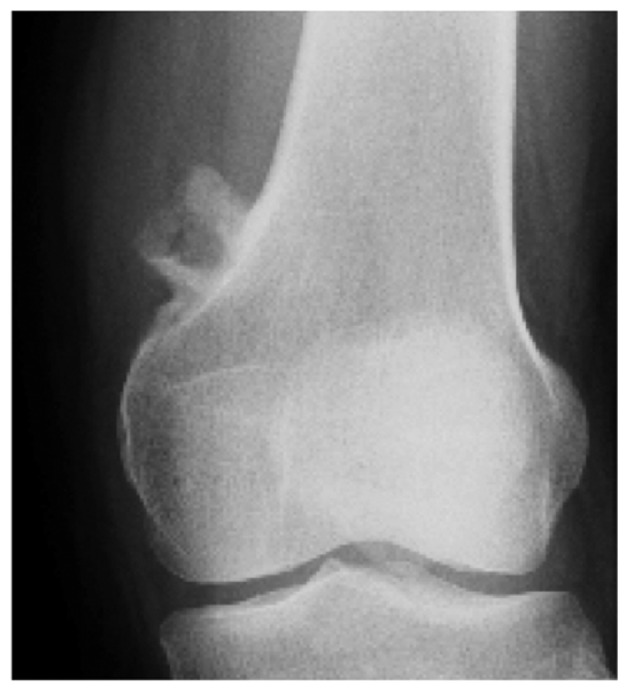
Anteroposterior radiograph revealing a well formed sclerotic periosteal reaction in the metaphysis of the distal femur.
